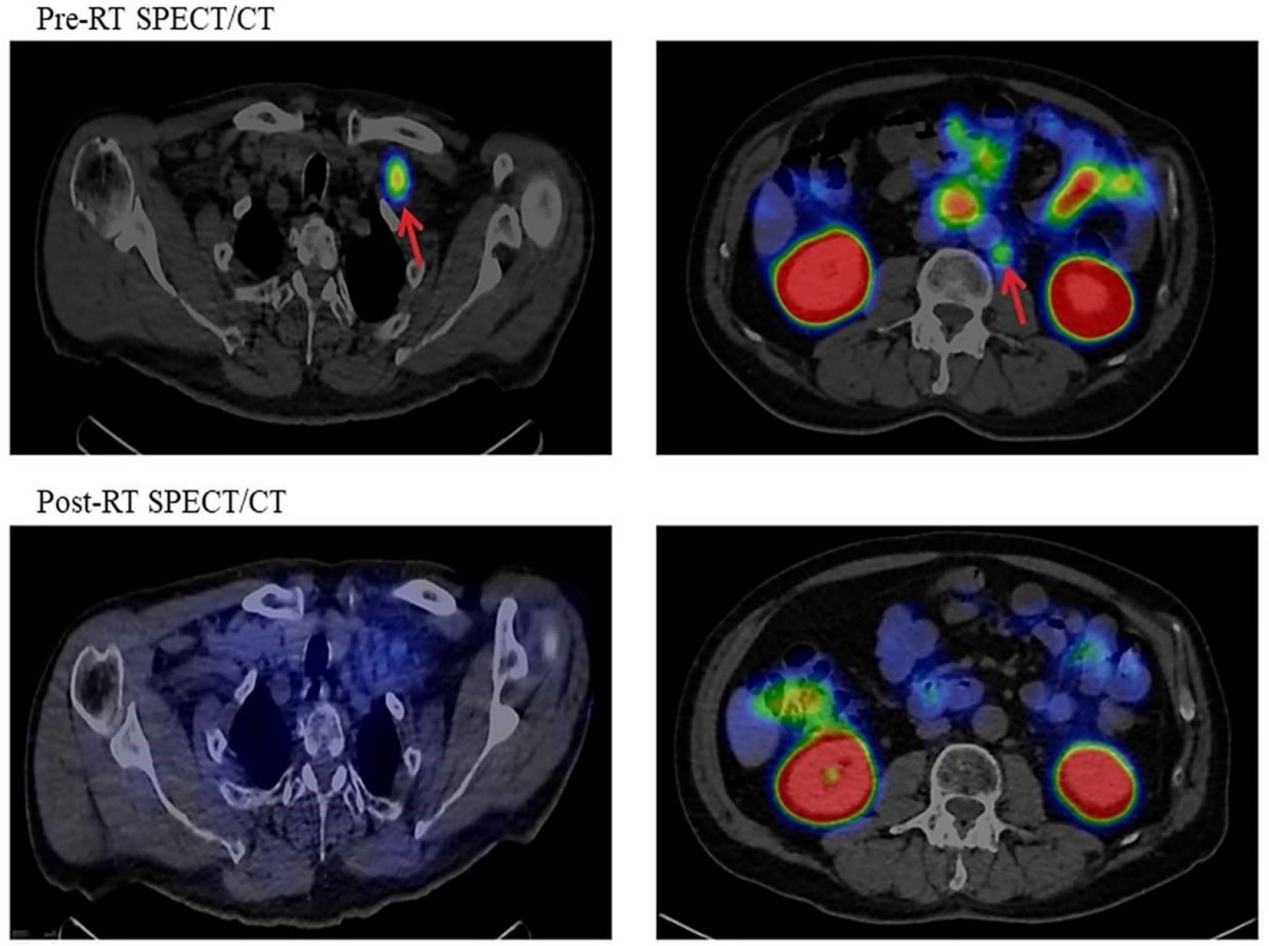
Pre- and post-RT PSMA SPECT/CT imaging. RT, radiotherapy; PSMA, prostate-specific membrane antigen; SPECT, single-photon emission computed tomography.
